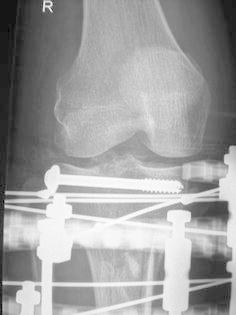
Post-operative radiograph showing limited internal fixation and circular external fixator
